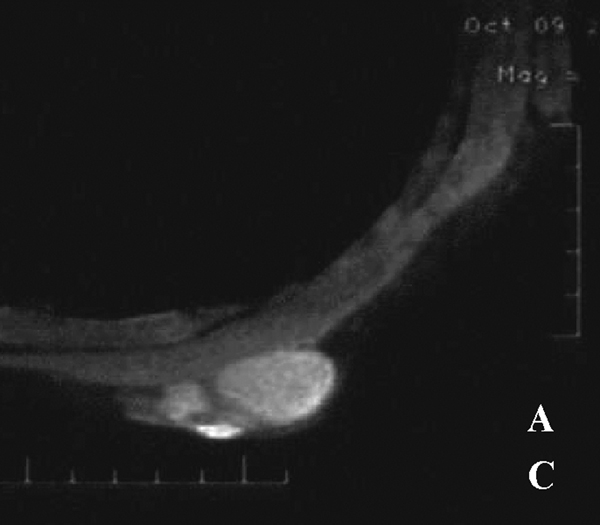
Axial fat-suppressed T1-weighted image shows two lesions clearly, and the signal intensity of both lesions is higher than that of the pectoralis major muscle.
